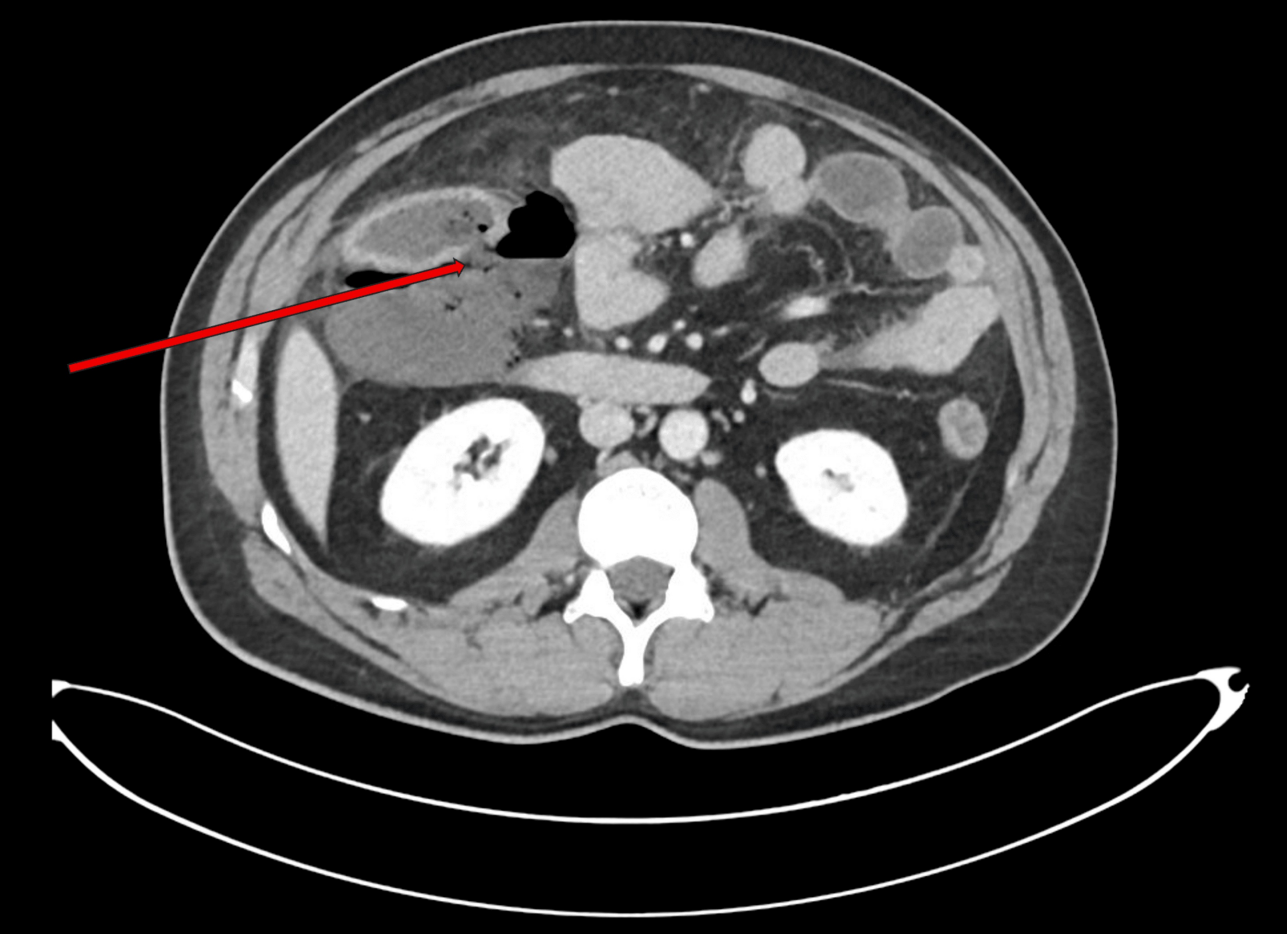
CT abdomen and pelvis with contrast axial view showing a focally dilated colonic loop with enhancement, colonic wall defect (red arrow), and extensive stranding and edematous changes in the adjacent peri-colonic mesentery and omentum, in keeping with secondary inflammatory changes.
